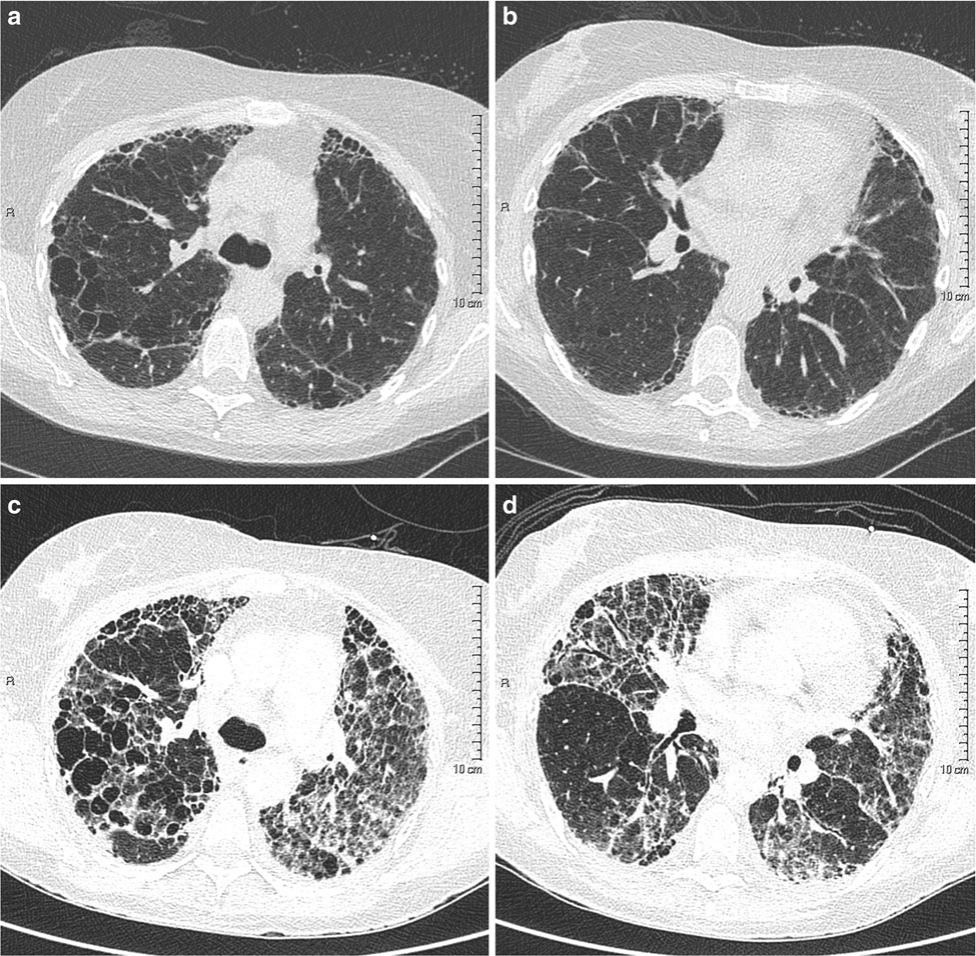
Serial chest HRCT images in relation to the suspected AE-IPF. HRCT performed during the exacerbation (c) and (d) demonstrates diffuse ground glass opacity with marked progression of the underlying fibrosis and honeycombing compared with 3 months prior (a) and (b).
